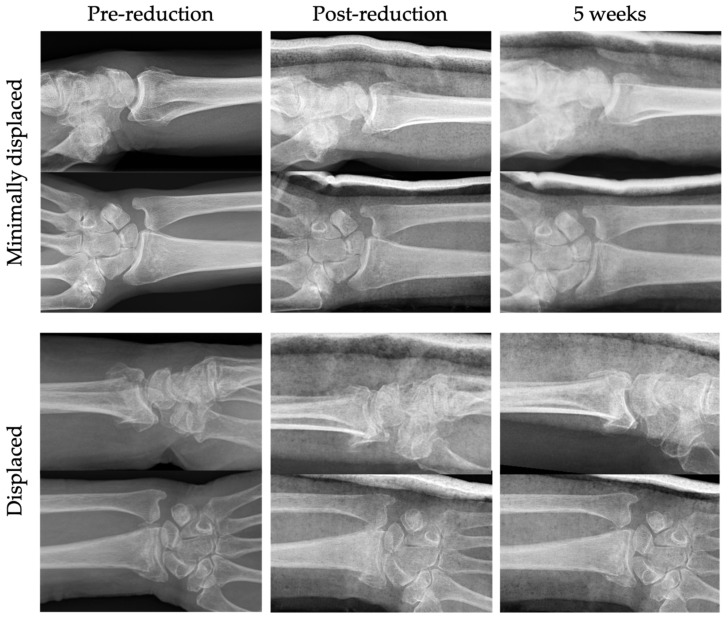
Examples of radiographs of the two non-operatively treated cohorts: minimally displaced distal radius fracture (DRF) and displaced DRF after two attempts of fluoroscopic guided closed reduction. Pre-reduction, post-reduction and 5 week follow up.
